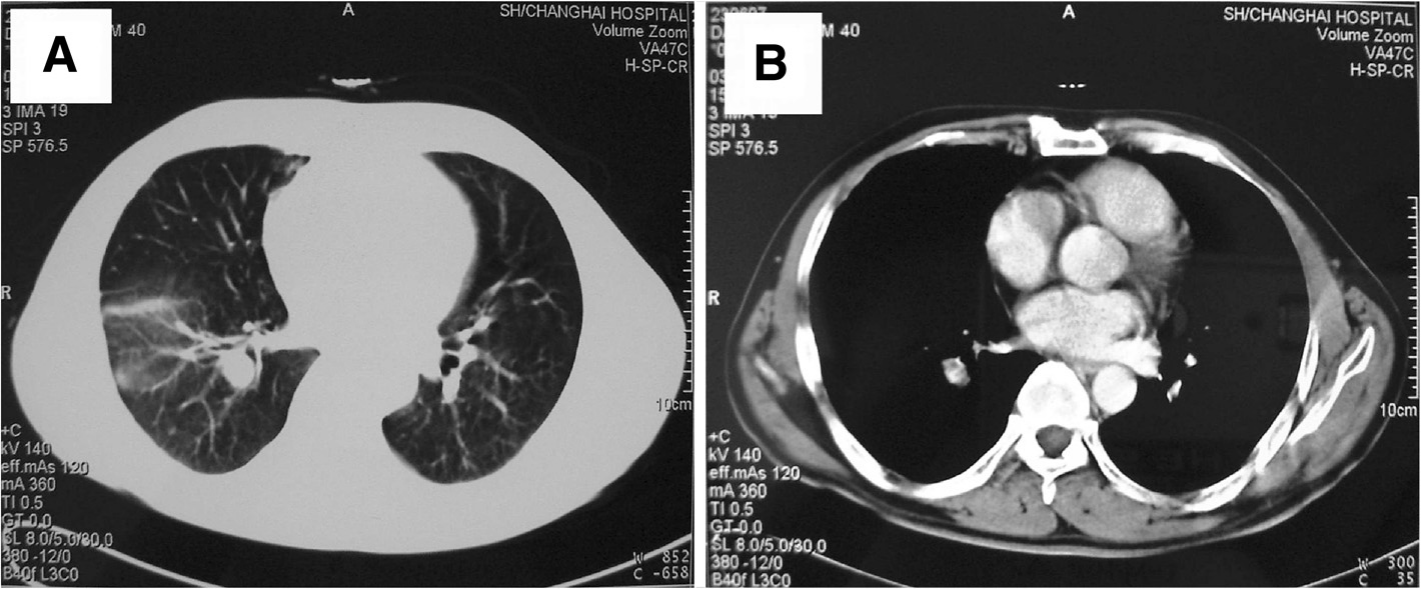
CT findings upon follow up.
